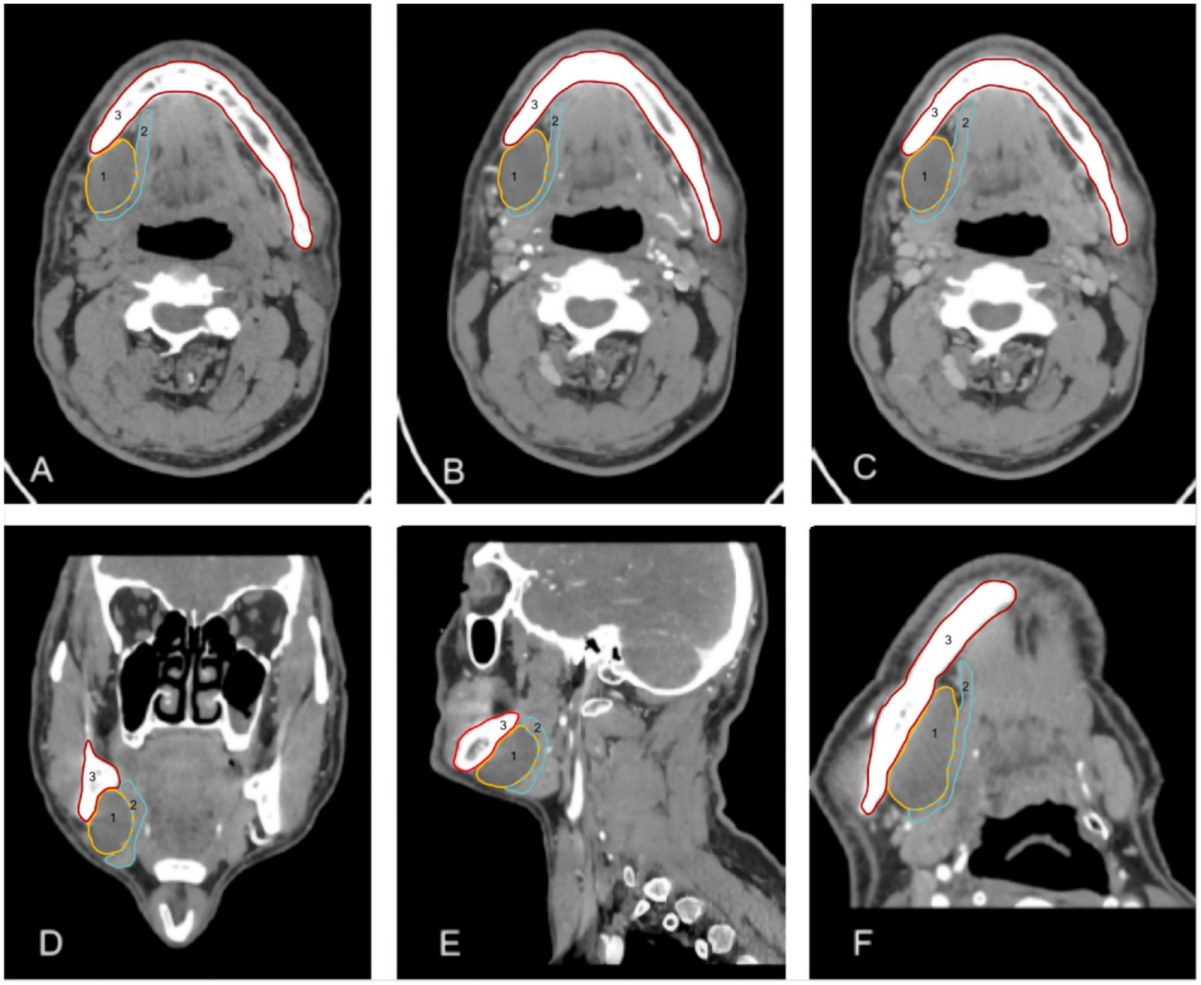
(A–C) represent the non-contrast, arterial-phase, and venous-phase CT scans of the salivary glands, respectively. (D) is a coronal reconstruction of the arterial phase, and (E) is an additional arterial-phase sectional image. (F) is a curved planar reconstruction image along the course of the right mylohyoid muscle in the arterial phase. The red area indicates the mandible, the yellow area denotes the lesion site, and the blue area represents the mylohyoid muscle.
